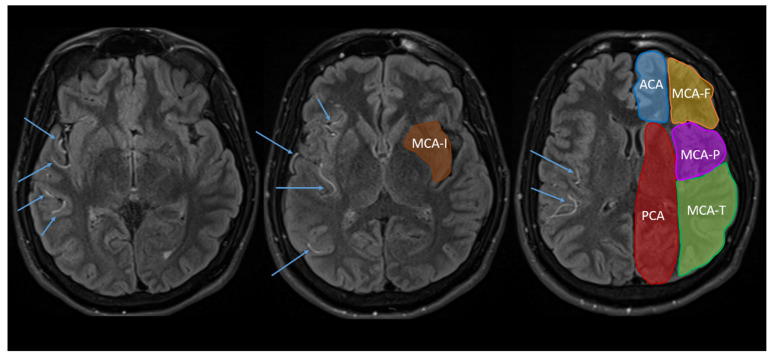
Examples of FHVs (indicated by the arrows) and vascular regions used for the NIH-FHV score: the anterior cerebral artery (ACA, blue), posterior cerebral artery (PCA, red), and four regions of the middle cerebral artery (MCA)—the MCA-frontal (MCA-F, orange), MCA-temporal (MCA-T, green), MCA-parietal (MCA-P, purple), and MCA-insular (MCA-I, brown). Note that no FLAIR imaging for the current study was shared by the NIH. Thus, this example of FHVs is from a participant in Bunker et al. [13].
